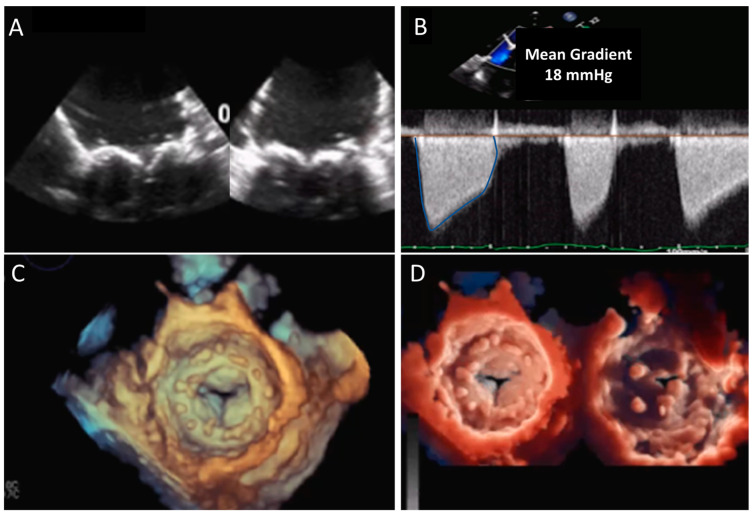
Biological mitral valve prosthesis degeneration. (A) TEE mid-esophageal views showing a marked thickening and degeneration of the MV prosthesis leaflets. (B)significant increase in the mean diastolic gradient of the prosthesis. (C) A 3D TEE surgical view of the MV prosthesis showing a marked reduction in the diastolic opening of the leaflets, as confirmed and further detailed by 3D TEE views from the LV or from the LA with the transillumination modality (D). MV, mitral valve; TEE, transesophageal echocardiography.
